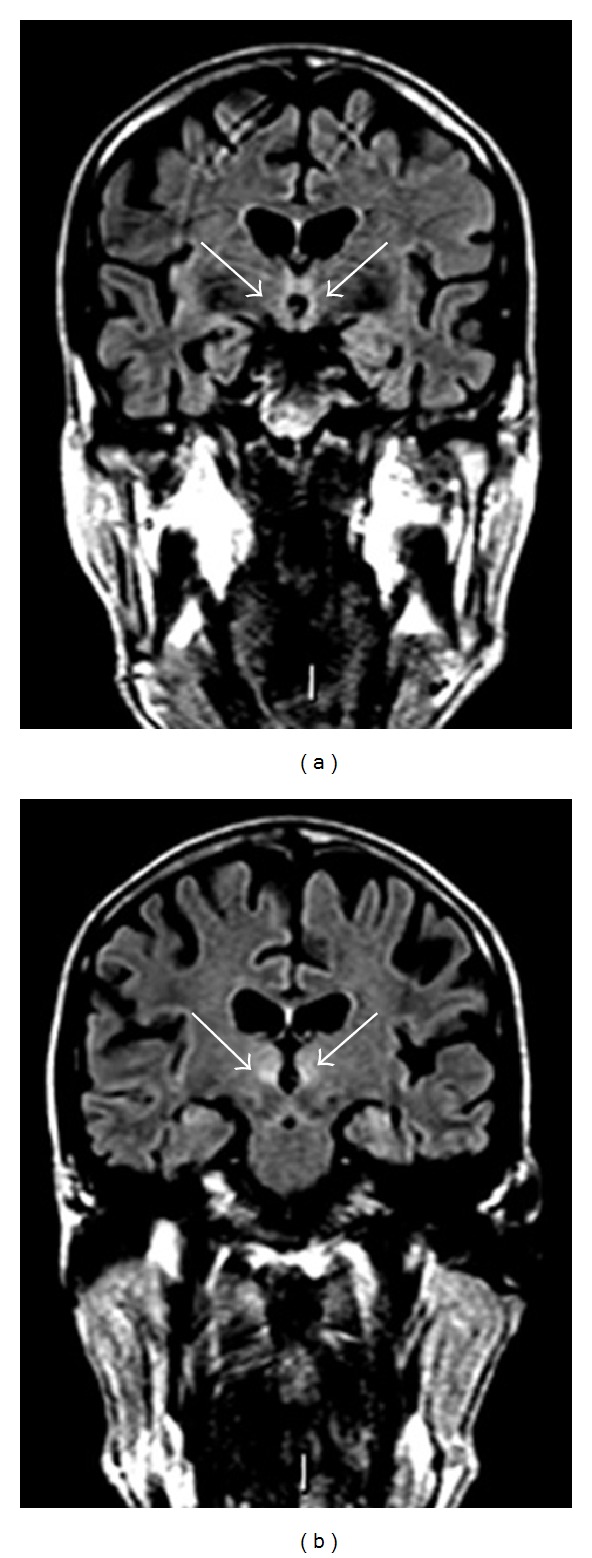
Fifty-year-old man with a 7-year history of alcohol abuse. FLAIR coronal images showing bilateral and symmetric hyperintense signal alteration at the level of the mammillary bodies (a) and the medial portion of the thalami (b).
